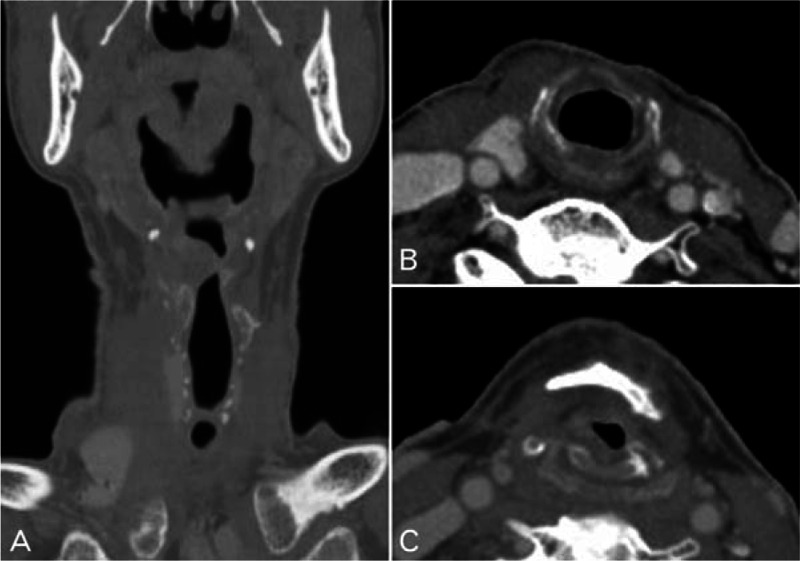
Contrast-enhanced computed tomography images 3 y after the second surgery. Images (A, B, and C) at bone windows show no signs of recurrence.
